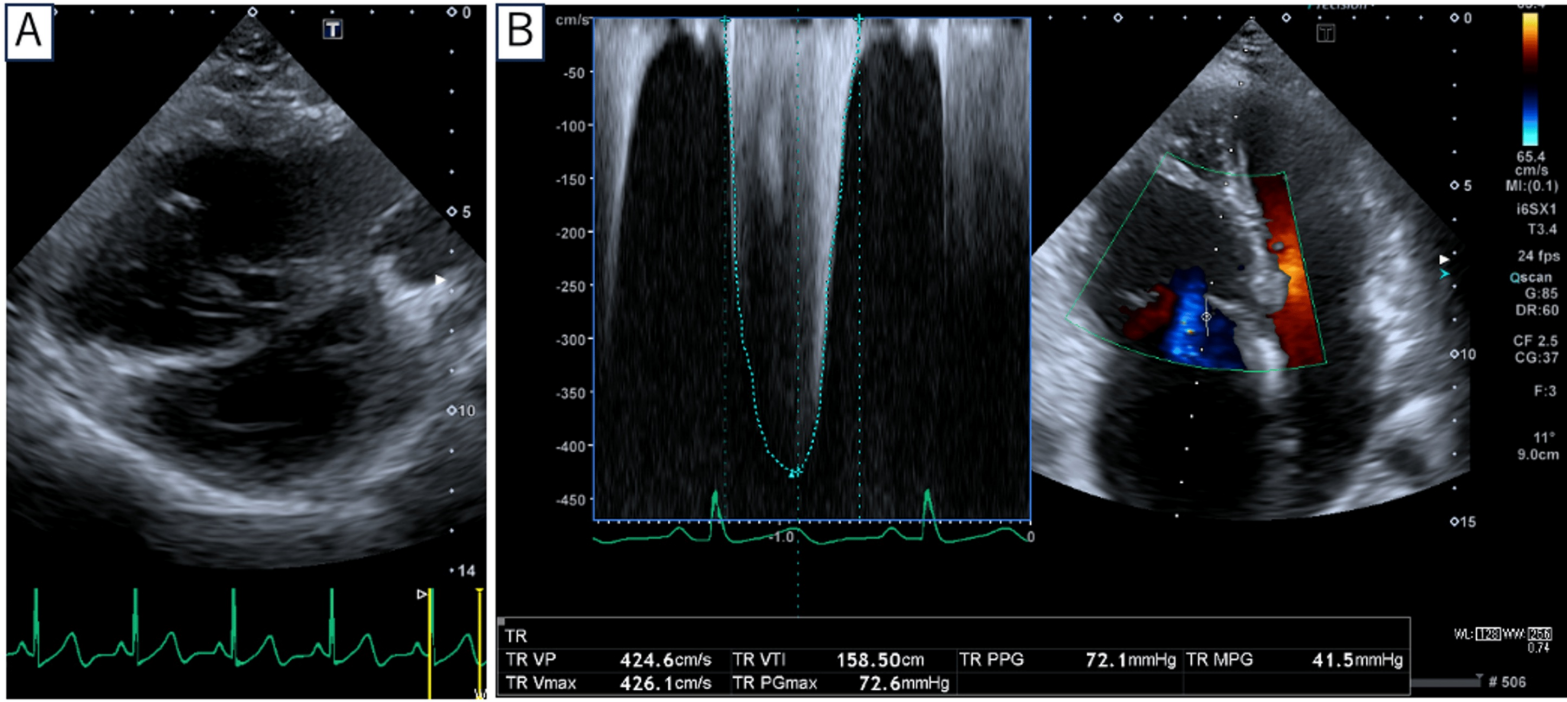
Transthoracic echocardiography. (A) Short-axis view demonstrating a D-shaped left ventricle. (B) Apical four-chamber view showing tricuspid regurgitation pressure gradient of 72 mmHg.
